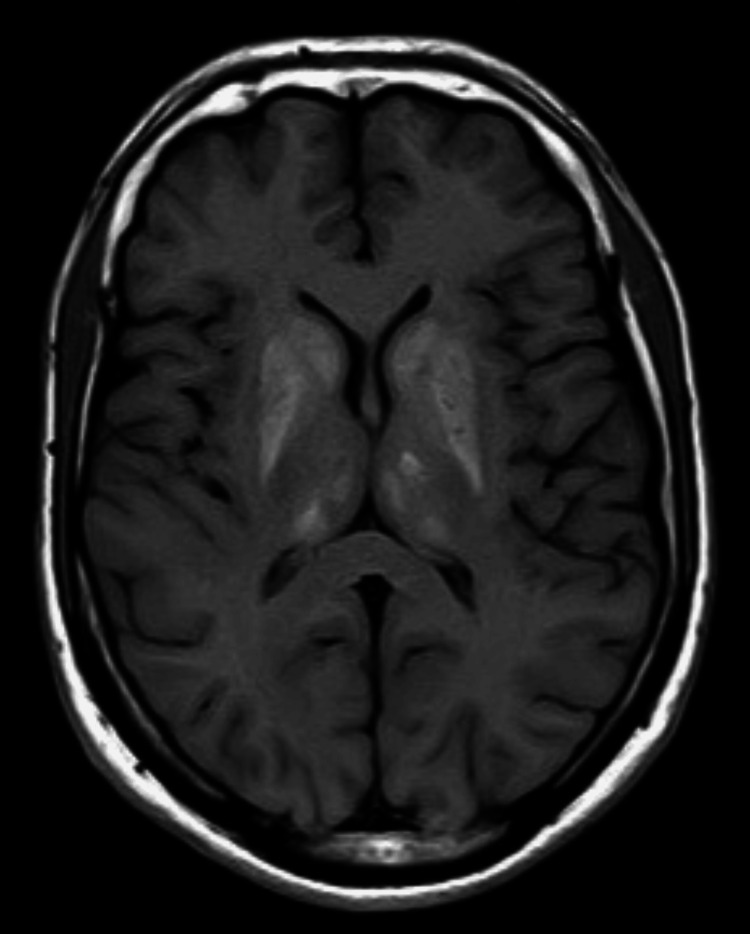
T1-weighted axial MRI of the brain (plain study) image at the level of basal ganglia showing hyperintense signal in the bilateral basal ganglia, with both thalami suggestive of calcification
